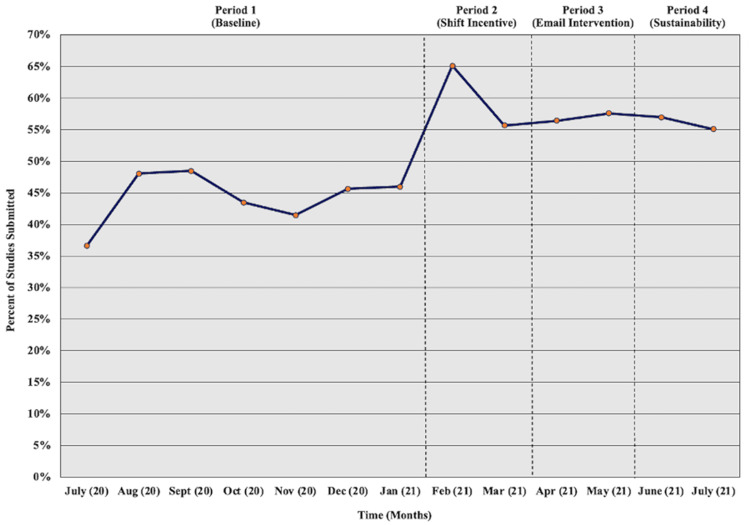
Percent of POCUS studies submitted per month for all eligible physicians combined over the study period (July 2020 – July 2021) POCUS, point-of-care ultrasound
